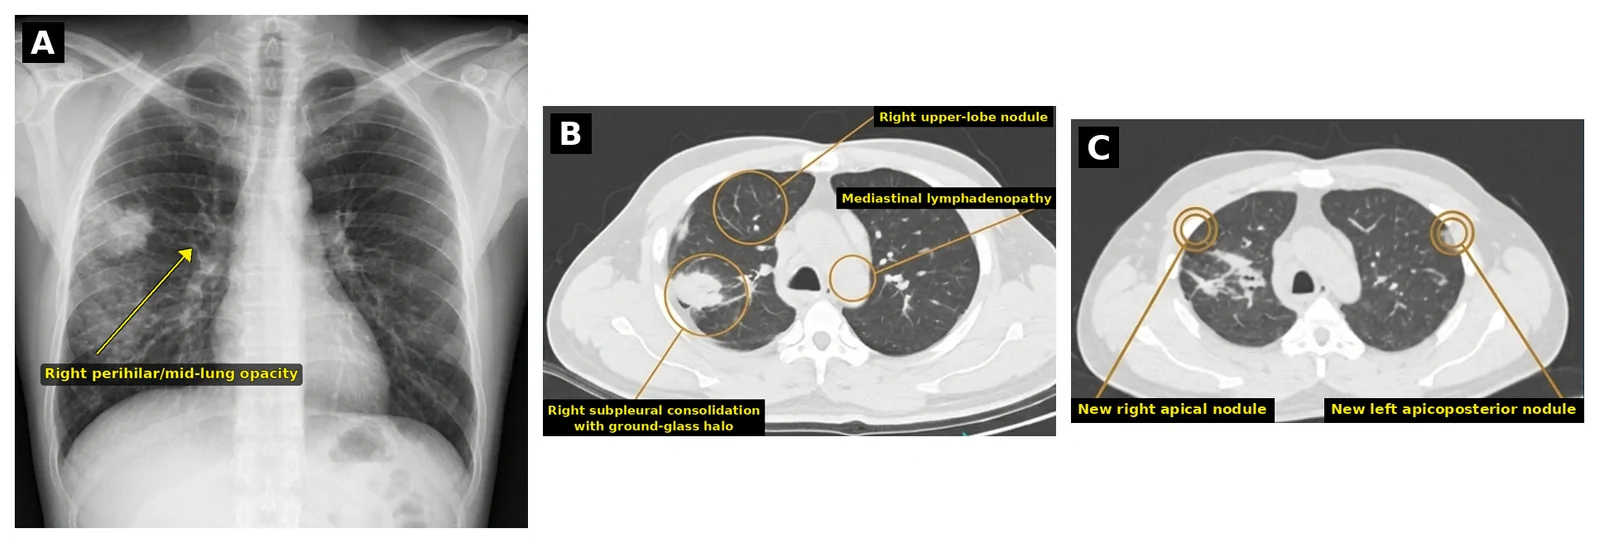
Serial thoracic imaging. (A) Posteroanterior chest radiograph at index presentation: a rounded right perihilar/mid-lung opacity (arrow). (B) Axial contrast-enhanced chest computed tomography (CT) at index presentation, showing a large right-sided subpleural consolidation with a peripheral ground-glass halo, a right upper-lobe nodule, and subcarinal lymphadenopathy; the circles mark these three findings and are labeled individually on the panel. (C) Axial contrast-enhanced chest CT at readmission, approximately five months later, showing interval regression of the original right-sided lesion with new bilateral pleural-based upper-lobe nodules (labeled arrows: a new right apical nodule and a new left apicoposterior nodule), consistent with a migratory pattern. Panels (B,C) are axial 1.25 mm sections from contrast-enhanced chest CT acquired on the same scanner using the same institutional protocol, displayed on a lung window (window width 1500 HU, window level −600 HU).
